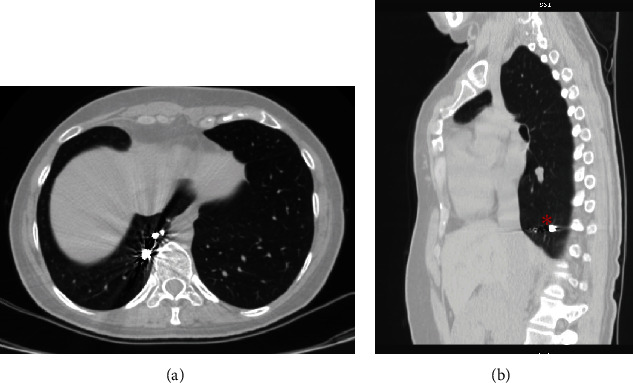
(a, b) Axial and sagittal views of the chest CT showing complete regression of the sequestered lobe with the embolization coils in place (red stars).
